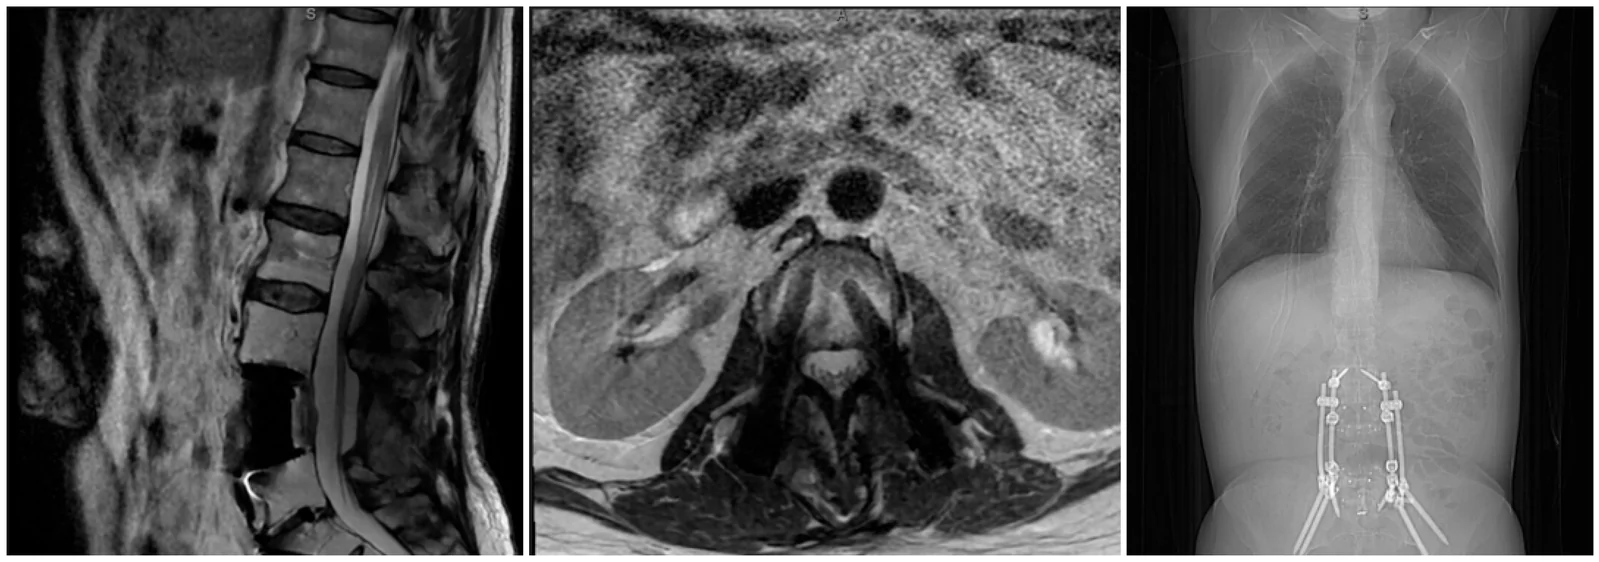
Postoperative MRI Lumbar Spine Sagittal, axial, with additional X-ray LRFS at 18 months follow-up.
